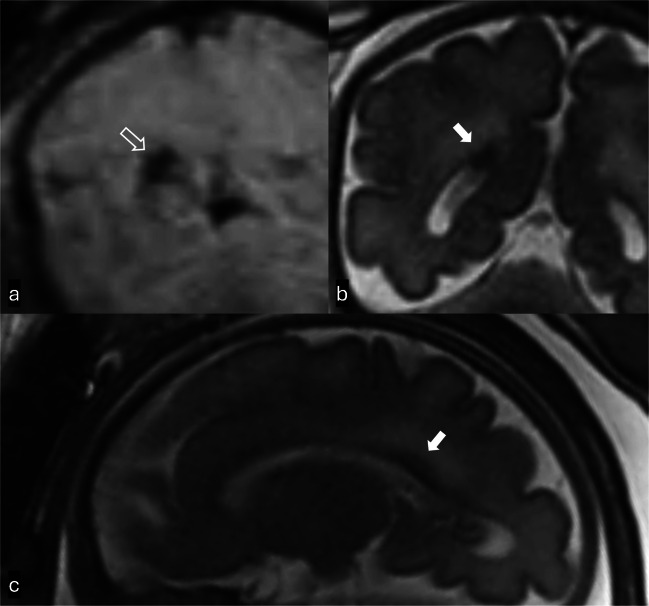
Magnetic resonance images of a developmental venous anomaly with deep drainage. Magnetic resonance images in a female fetus (#15) at 33 weeks’ gestational age demonstrate parieto-occipital developmental venous anomaly and deep drainage. a Coronal susceptibility-weighted image shows blooming artifacts corresponding to the developmental venous anomaly (*empty arrow*). b Coronal T2-weighted images showing hypointense lesions “enveloping” the superior margin of the right lateral ventricle at the level of the atrium (*arrow*). c Parasagittal image demonstrating small hypointense lesions lining the ventricular wall (*arrow*)
